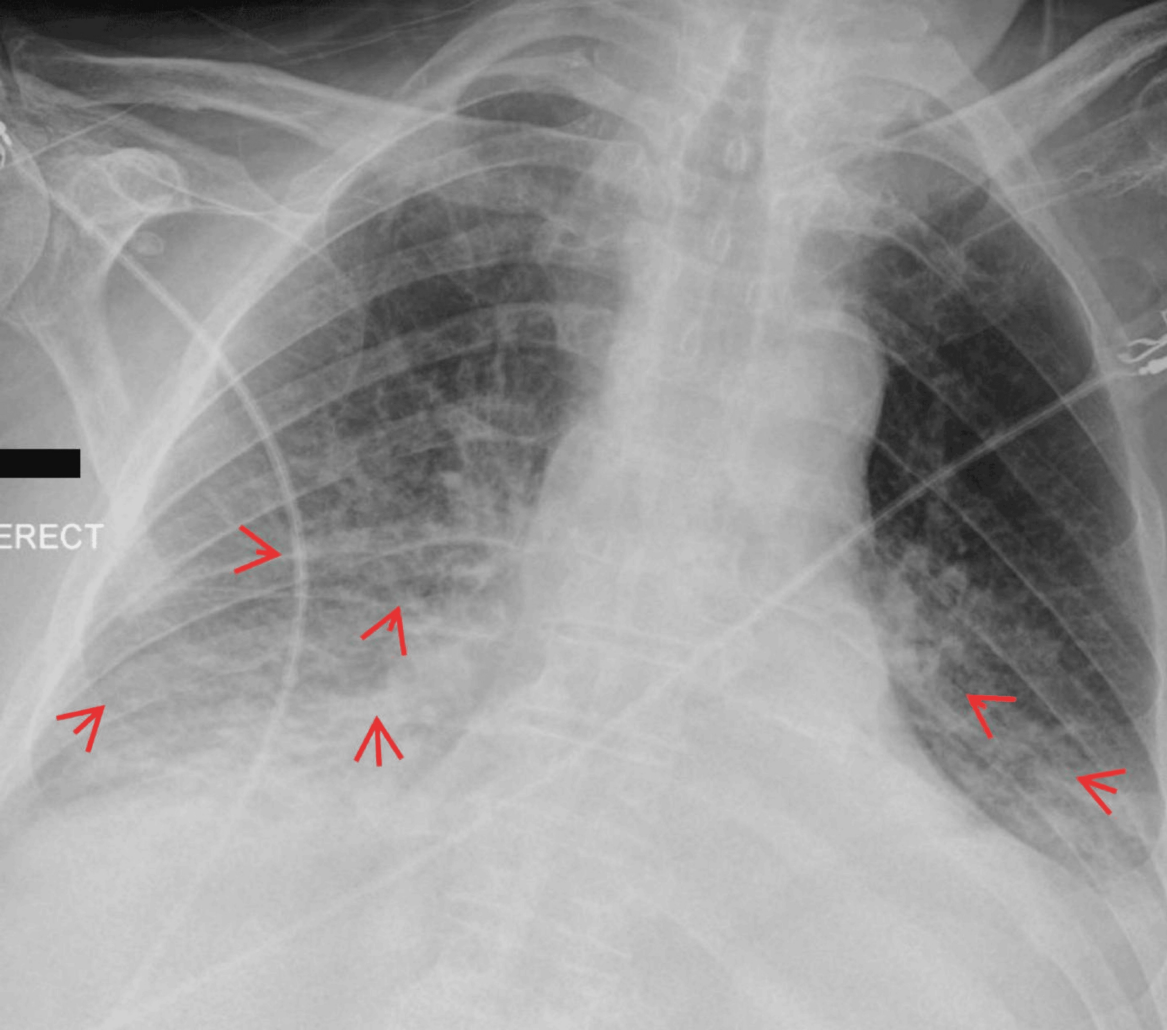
Chest X-ray indicating progressive bilateral opacifications (red arrows)
